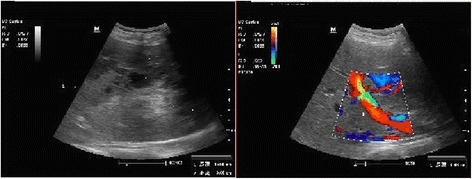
Ultrasound scan of the digestive system. A heterogeneous high echogenic mass can be seen in the right liver lobe. An irregular anechoic area is also evident in the mass; color Doppler flow imaging showed no obvious blood signal in it
